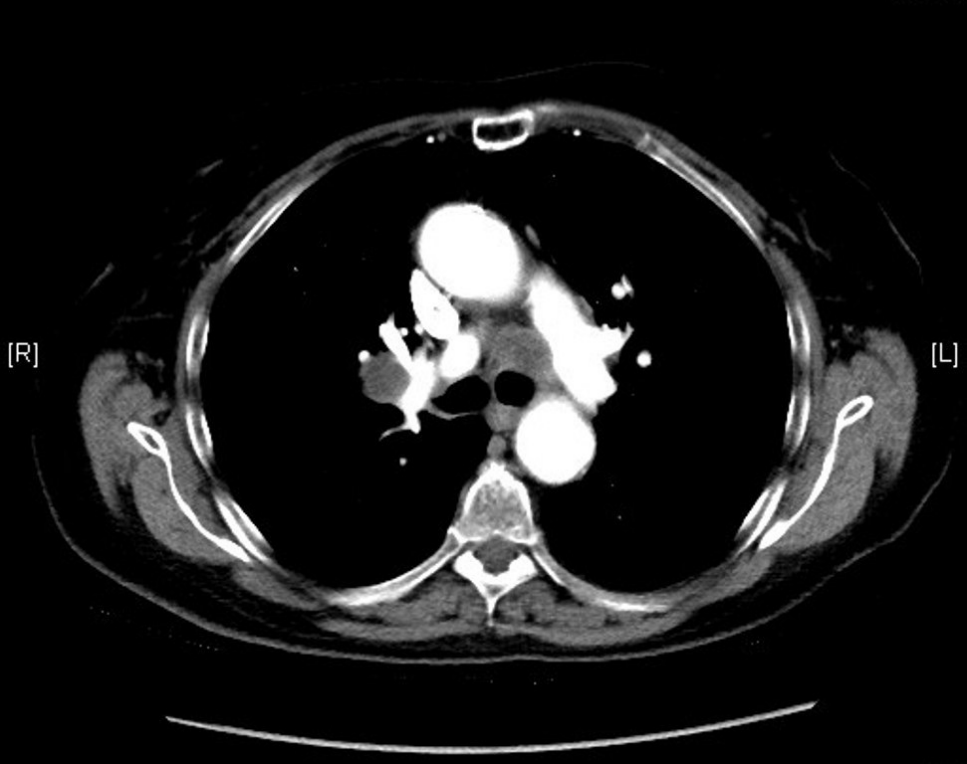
CT scan of the chest from 2015 showing precarinal adenopathy
